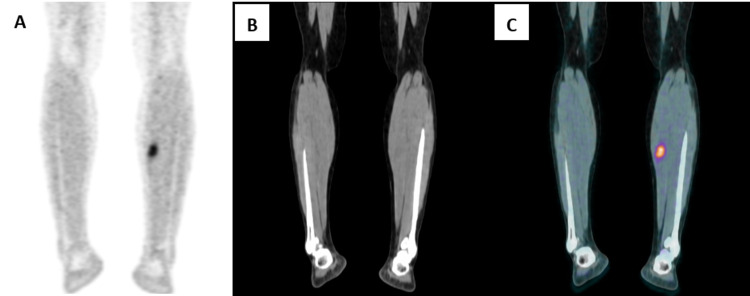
Coronal slices of the FDG PET-CT of the leg region. (A) PET images of the legs, revealing a hypermetabolic focus in the left leg. (B) CT component of the PET-CT showing a small hypodense lesion in the left soleus. (C) Fused PET-CT image showing intense FDG uptake in the left soleus corresponding to the hypodense lesion appreciated on the CT. CT: computed tomography; FDG: 18F-fluorodeoxyglucose; PET: positron emission tomography
